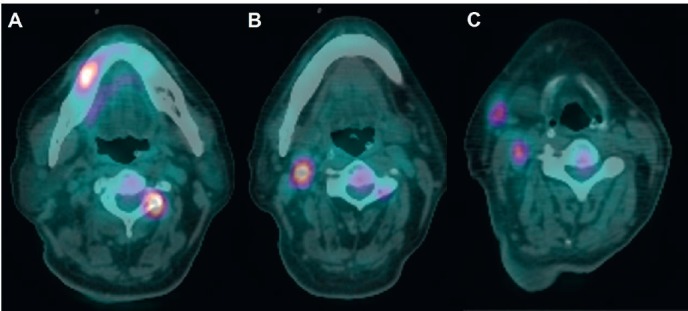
PET/CT scan. (A) A 1.8-cm lesion with moderate uptake over right lower buccal/gingival area with an avid metastatic lesion over spine. (B and C) Lymph node metastases over right neck.
